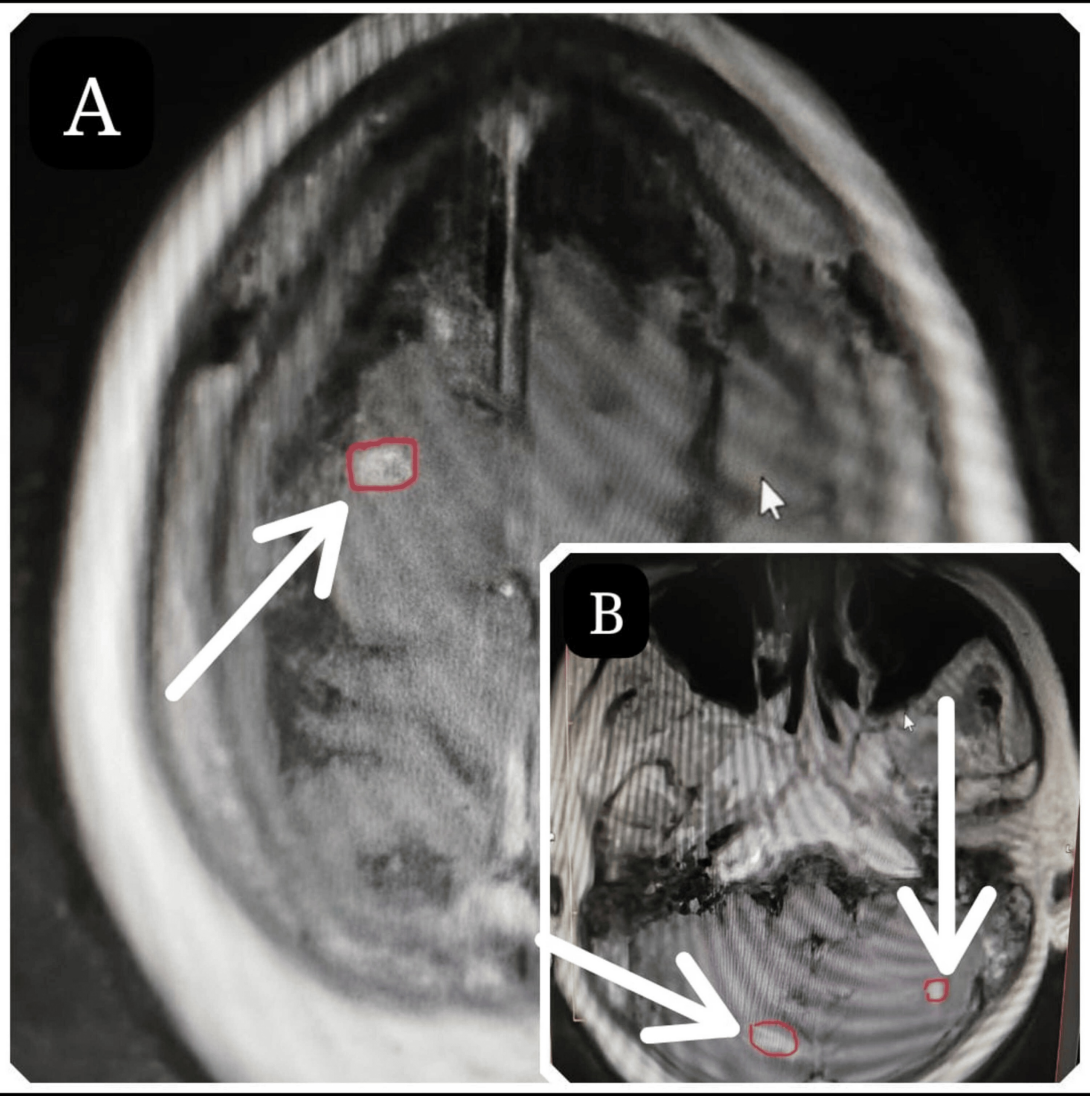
Contrast-enhanced MRI of the brain at the fourth month of follow-up showing right frontal (A) and bilateral cerebellar (B) metastatic deposits (white arrows) from the primary left breast invasive ductal carcinoma.
